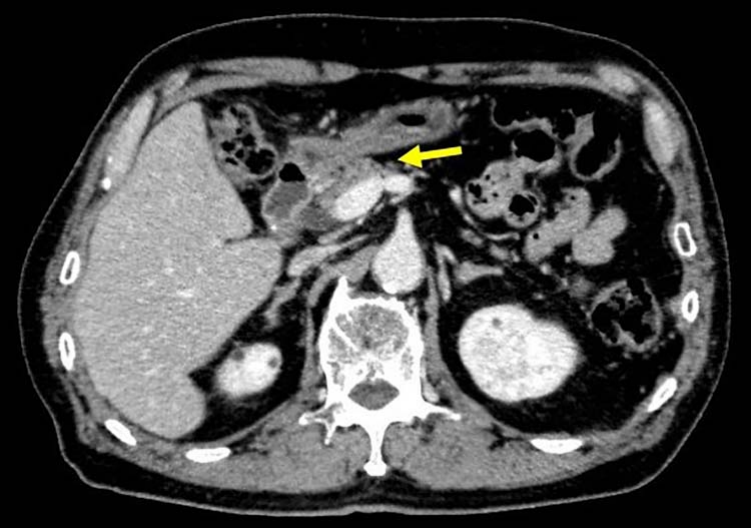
CT after the NAC had been completed showed a significantly shrunken tumor at the antrum that had become detached from the pancreatic head (arrow).
